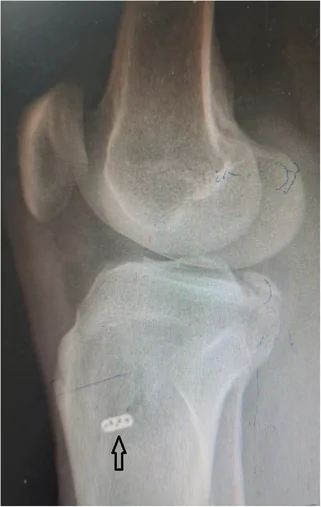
The arrow points to the tendon line fixed with a button plateon the outer side of the tunnel as seen under fluoroscopy, using 1 to 2 button plates as needed. (Right knee, lateral radiograph).
